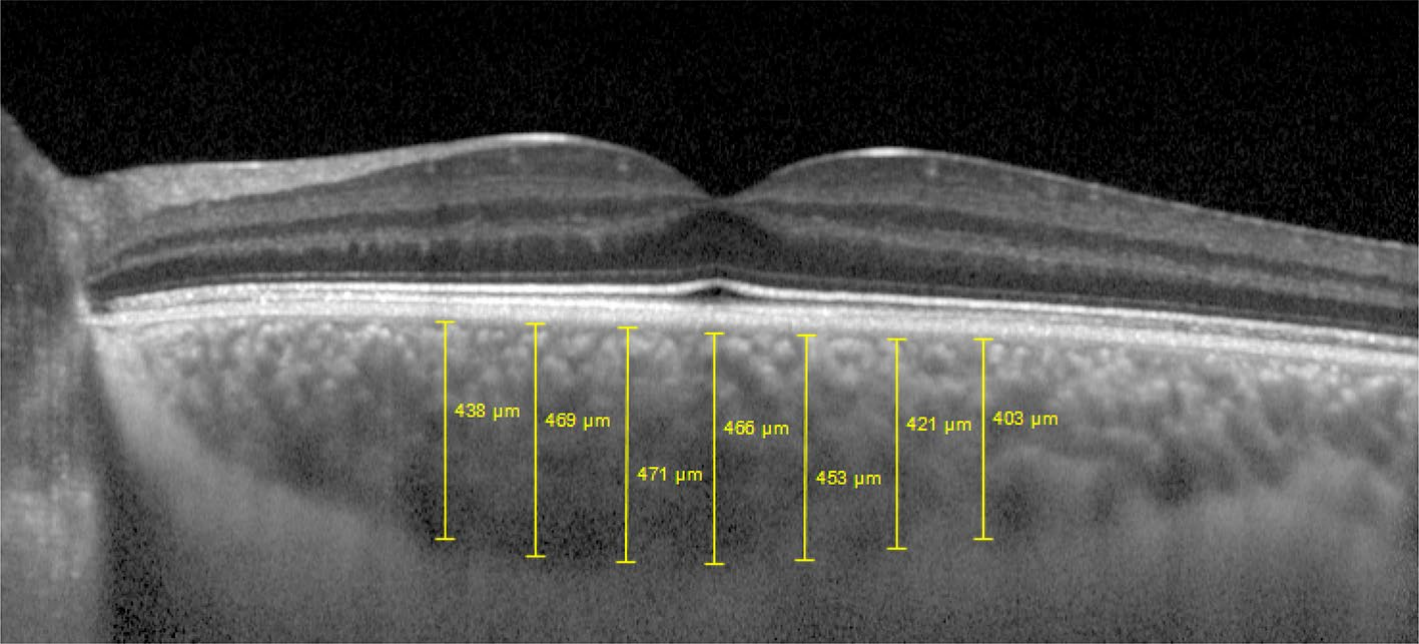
Representation of a CT measurement in a KC eye using the semiautomatic mode. Measurements were taken at the subfoveal choroid and at 500 µm intervals from the fovea, temporal 500 μm, 1000 μm, 1500 μm, and nasal 500 μm, 1000 μm and 1500 μm.
